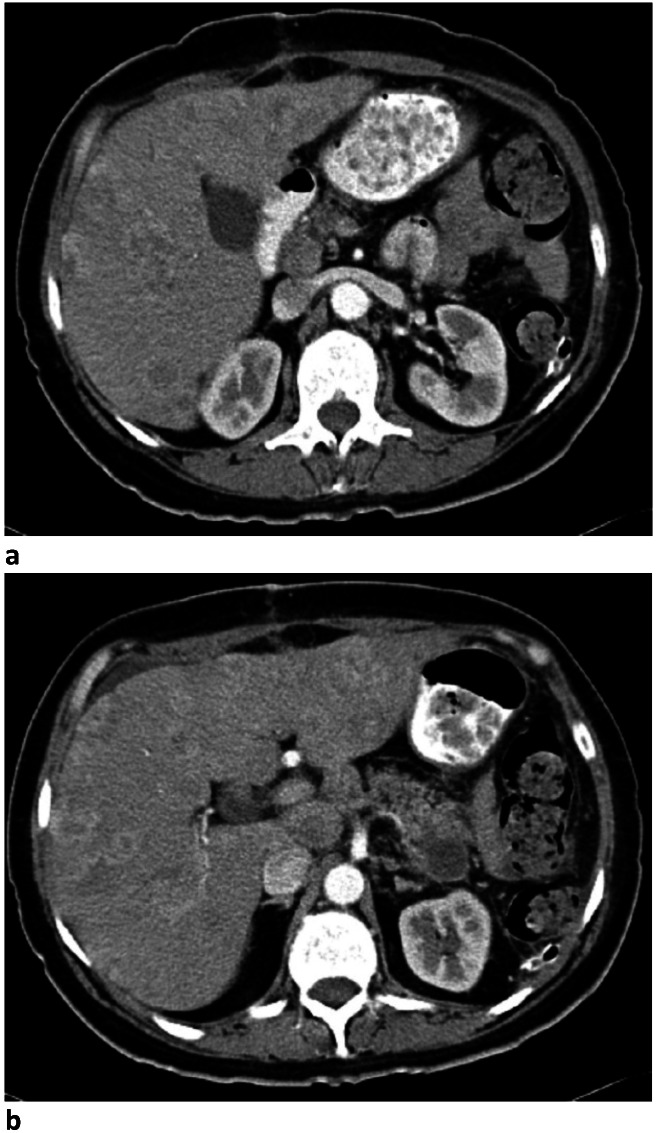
Computed tomography location and aspects of pancreatic tumor a,b
